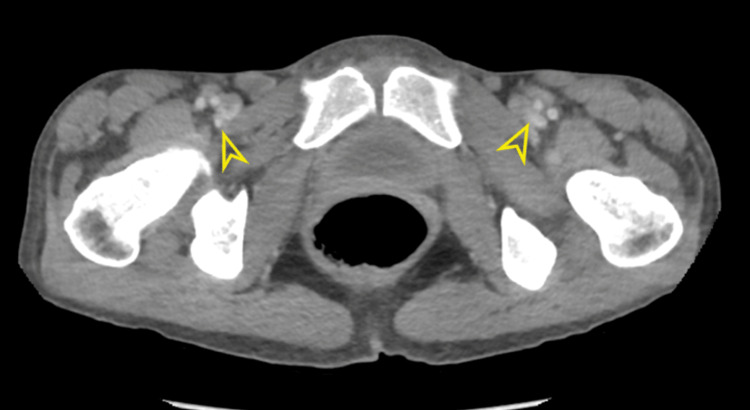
Selected image of axial contrast-enhanced abdominal CT scan showing multiple prominent bilateral inguinal lymph nodes.
